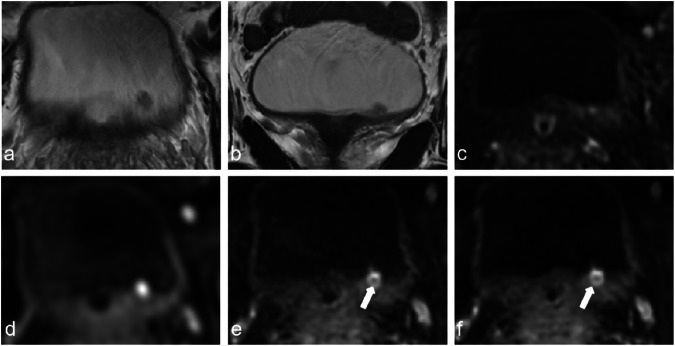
A 69-year-old man with pTa high-grade urothelial carcinoma. Axial (a) and coronal (b) T2WI show a small exophytic tumor on left lateral bladder wall with continuous low-signal intensity muscularis propria. DCE (c) image shows early enhancement of tumor. fFOV DWI (d) shows a high signal-intensity tumor without stalk. rFOV_STA_ DWI (e) and rFOV_DLR_ DWI (f) show high signal-intensity tumor with a low-signal-intensity stalk (arrow). All sequences were assigned category 1 and the VI-RADS score of all sets was 1. DCEI, dynamic contrast-enhanced imaging; DWI, diffusion-weighted imaging; fFOV, full field-of-view; rFOV, reduced field-of-view; rFOV_DLR_, rFOV with DLR; T2WI, T2-weighted imaging
